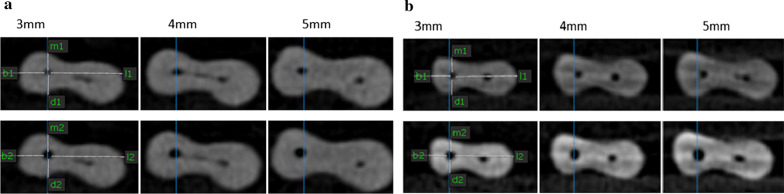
(a). CBCT scans before and after instrumentation of mesiobuccal canal by XP-endo Shaper at 3, 4 and 5 mm from the apex. (b) CBCT scans before and after instrumentation of mesiobuccal canal by ProTaper Universal at 3, 4 and 5 mm from the apex
